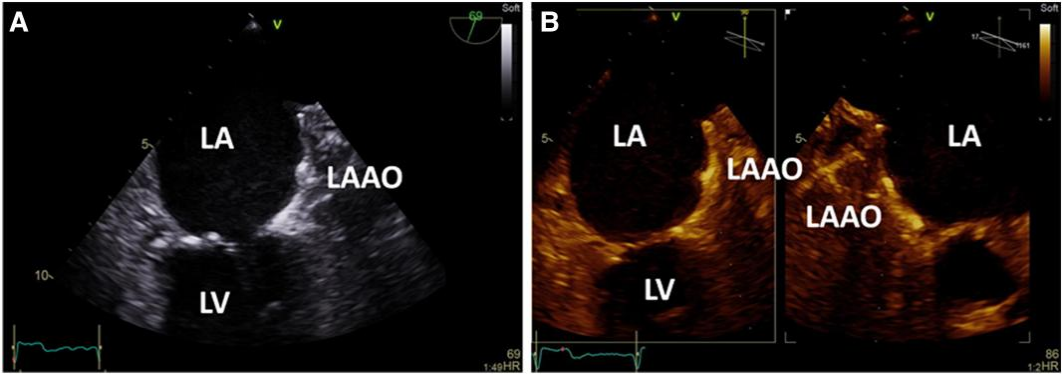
Follow-up transoesophageal echocardiography (TOE) after anticoagulation therapy. TOE demonstrating complete resolution of thrombus on left atrial appendage occlusion (LAAO) on grayscale map (*A*) and B-mode colorization (*B*). LA, left atrium; LV, left ventricle.
